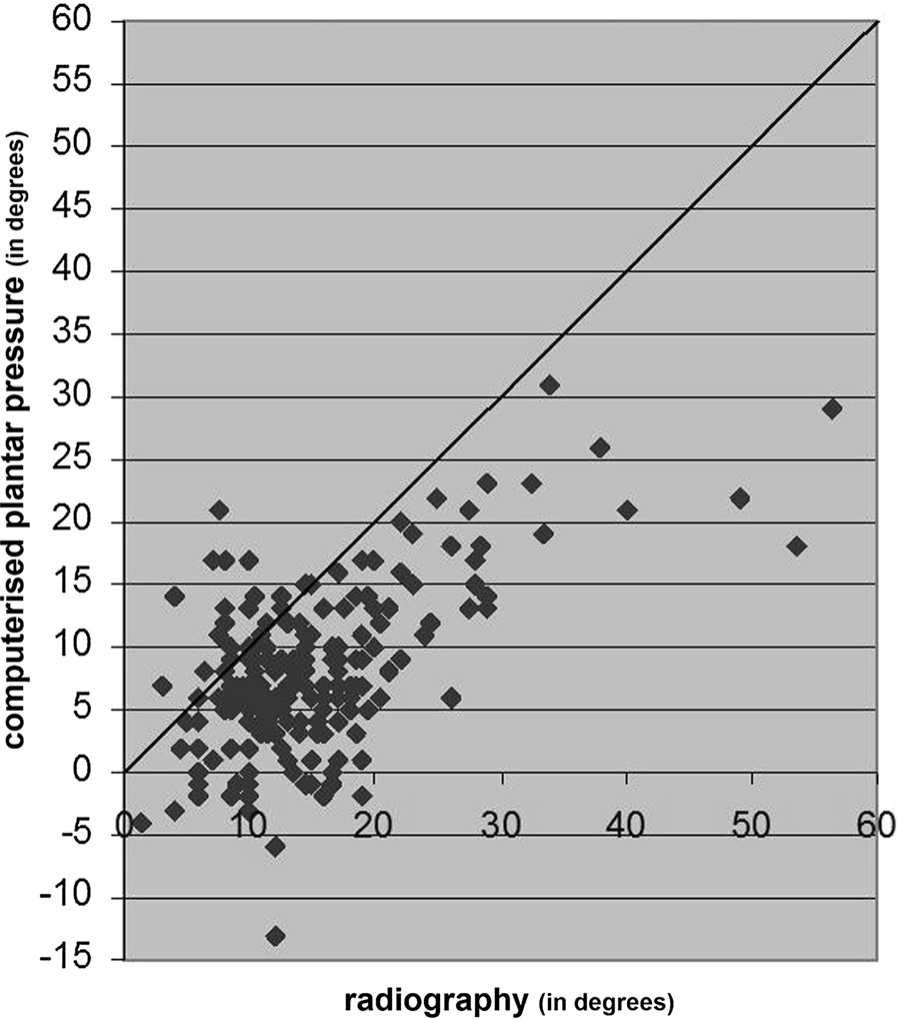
The results of computerised plantar pressure measurement of HVA compared with the results of radiographic measurement of HVA.
